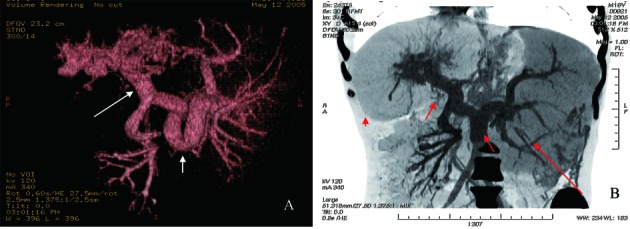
A: CT angiography of visceral vascular, tortuous thickening spleen vein (short white arrow) and proximal portal vein (long white arrow); B: Reconstruction of CT angiography, hepatomegaly (red arrowhead), splenomegaly (long red arrow), circuitous thickening spleen and portal vein (short red arrow).
